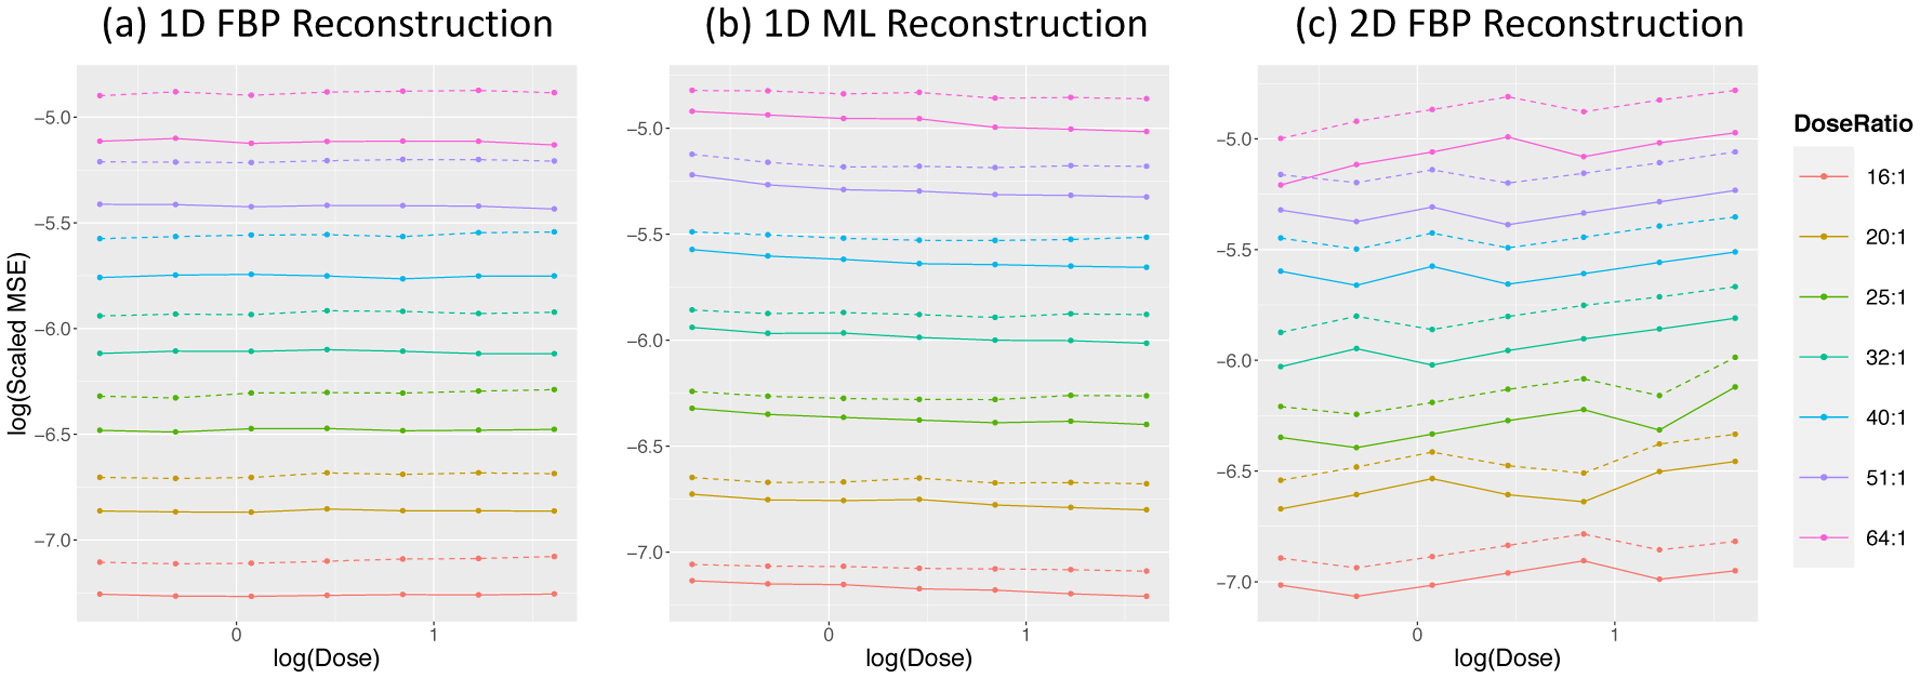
MSE values scaled by image data accuracy for combined (solid) and separate (dashed) analysis as a function of dose and dose ratio in H_2_O-FDG study.
